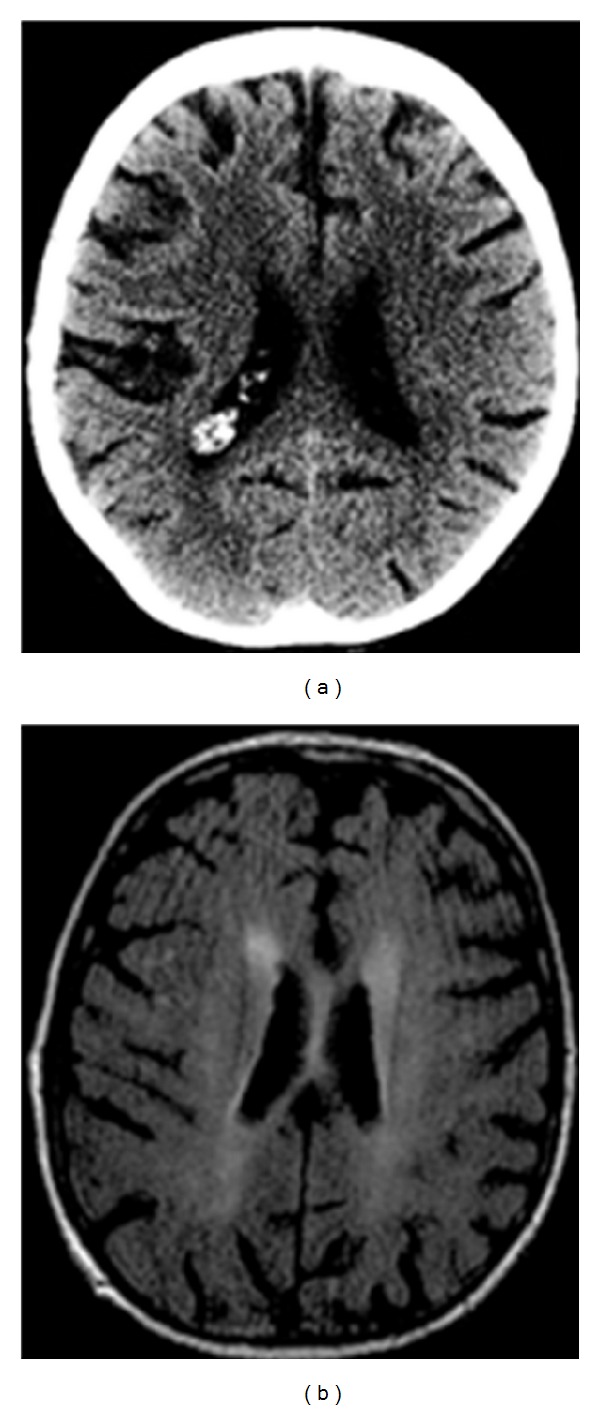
Associated findings. Unenhanced CT and MR FLAIR images of the brain demonstrate non-specific white matter related changes that can be associated with peripheral hemorrhages in cerebral amyloidosis.
